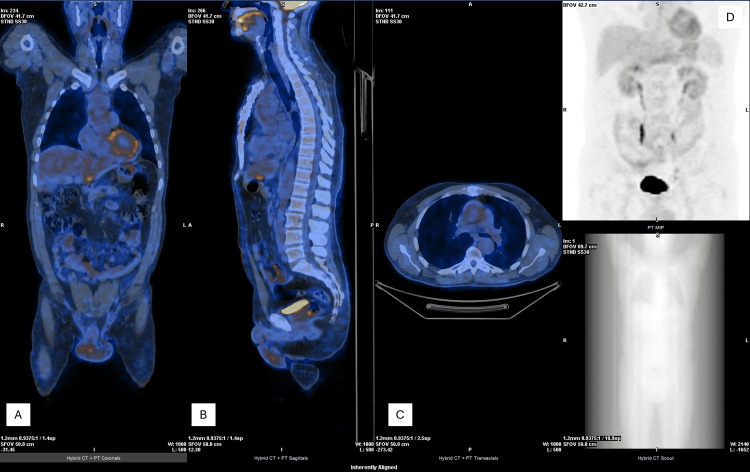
18F-FDG PET/CT imaging. Coronal (A), sagittal (B), axial (C), and MIP (D) PET/CT images showing no residual inflammatory uptake, consistent with metabolic remission. 18F-FDG, 18F-fluorodeoxyglucose; CT, computed tomography; MIP, maximum intensity projection; PET, positron emission tomography
